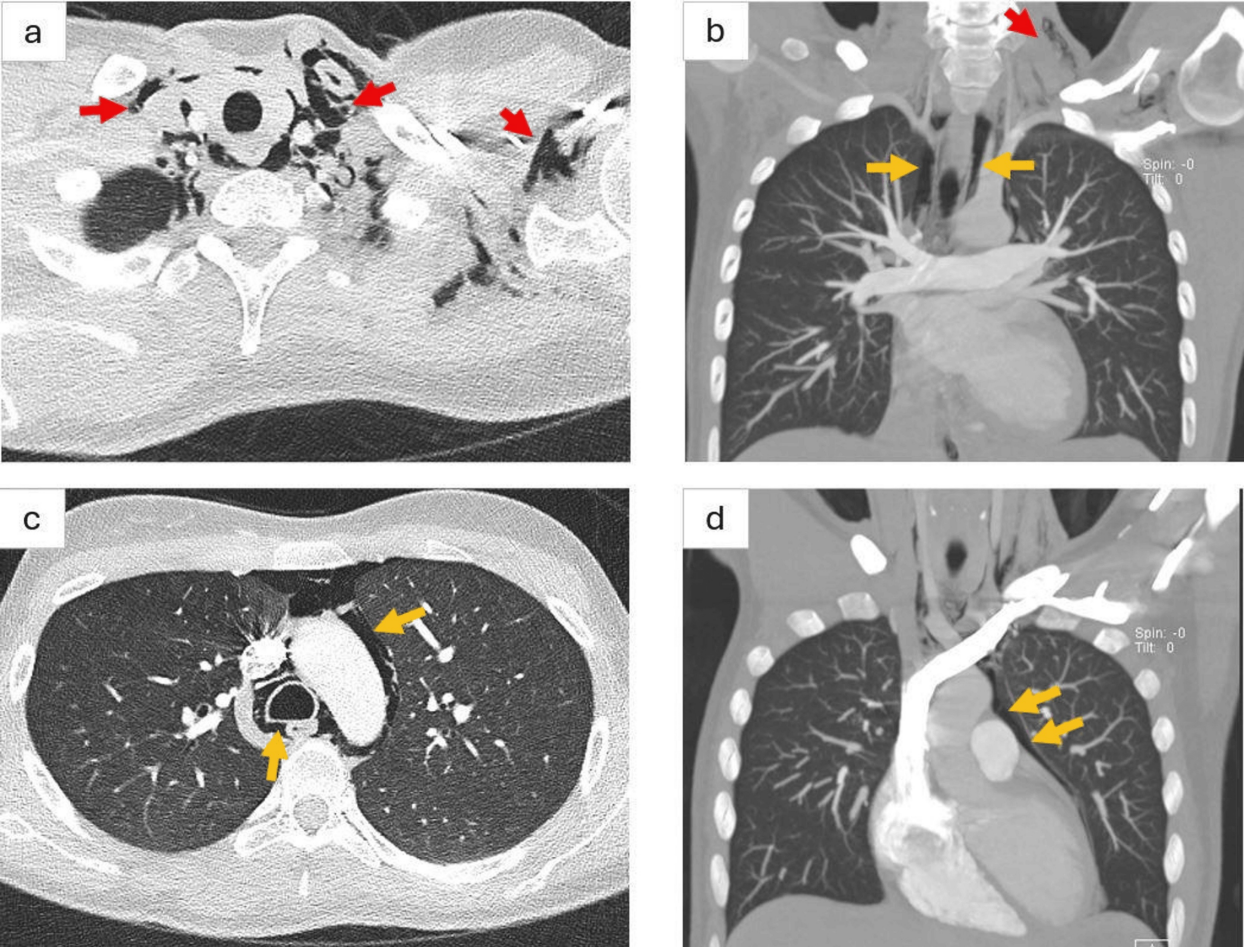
Intravenously enhanced computed tomography pulmonary angiogram. Selected images of axial (a, c) and coronal (b, d) planes of intravenously enhanced computed tomography pulmonary angiogram (lung window) revealed significant subcutaneous and inter-fascial air density seen on both sides of the lower neck (red arrows) and the left axilla. Further air density is seen at the mediastinum and along the azygoesophageal recess (orange arrows). Features in keeping with surgical emphysema and pneumomediastinum.
